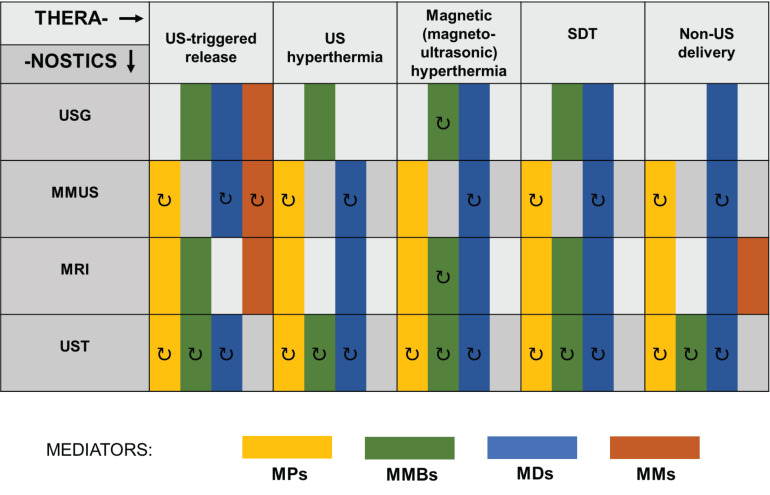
Combinations of imaging and therapeutic techniques involving US and various types of magnetic mediators. MDs, magnetic droplets and liposomes; MMs, magnetic microrobots and other vehicles; MMBs, magnetic microbubbles; MMUS, magnetomotive ultrasound imaging; MPs, magnetic particles; MRI, magnetic resonance imaging; SDT, sonodynamic therapy; US, ultrasound; USG, ultrasonography; UST, ultrasonic tomography. Proposed theranostic perspectives that could emerge in the future are indicated with the symbol ↻.
